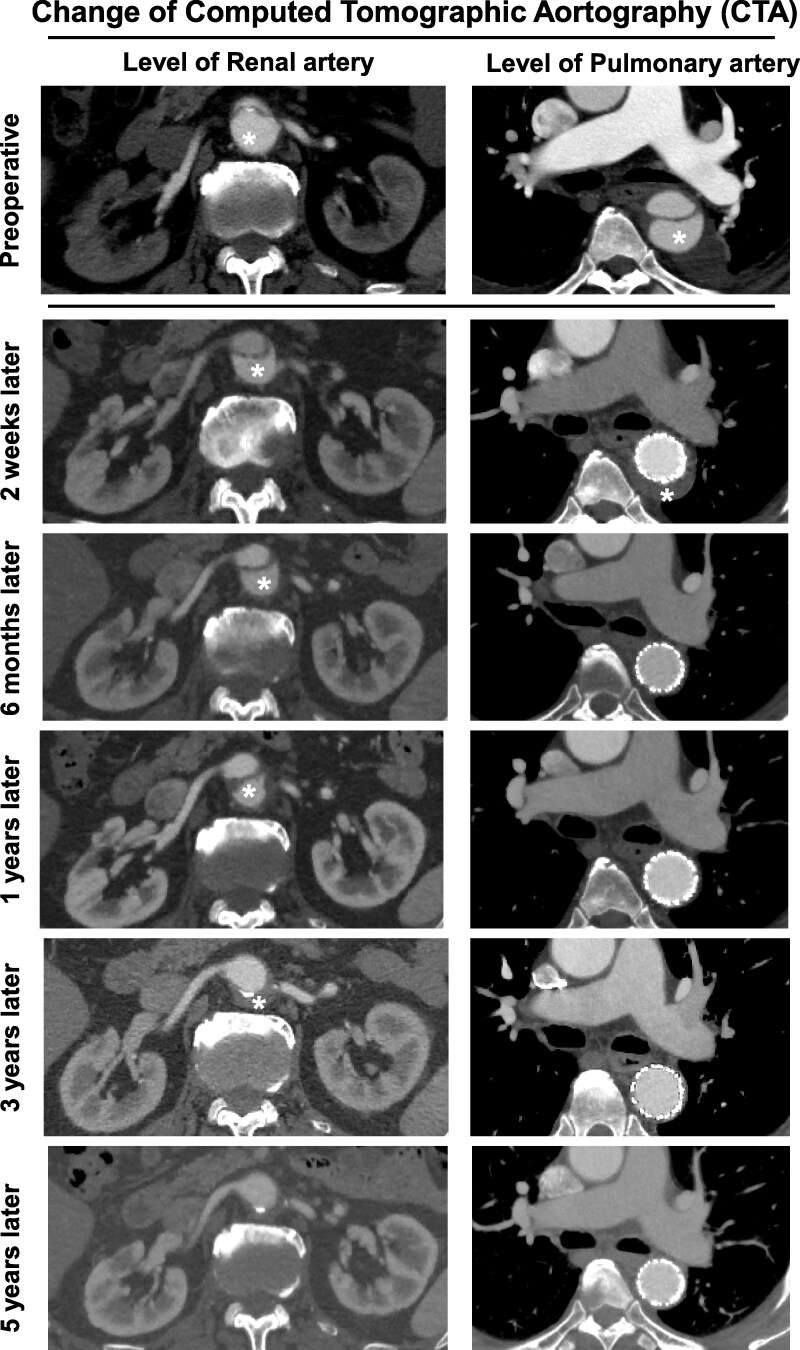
Preoperative and postoperative computed tomography findings. An asterisk (*) indicates the false lumen. (Left) Computed tomography images at the level of the renal artery. (Right) Computed tomography images at the level of the pulmonary artery.
